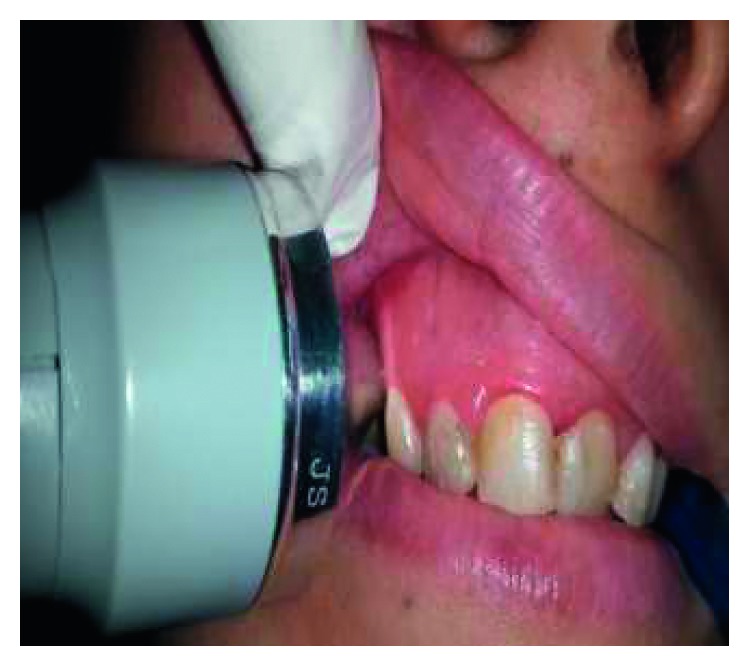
Ultrasound therapy delivered using probe on the buccal aspect of the implant site.
